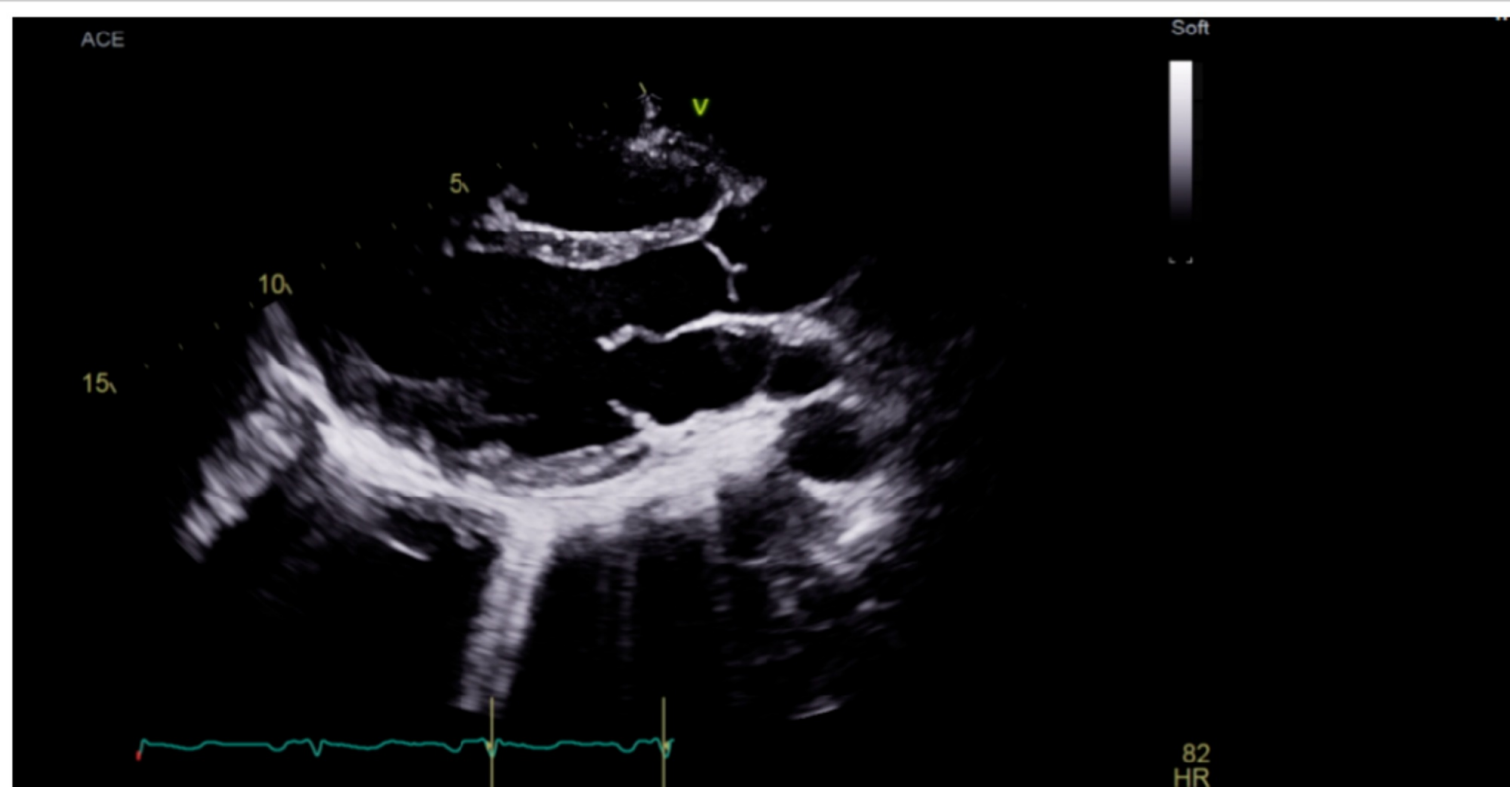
Transthoracic echocardiogram showing no vegetation
